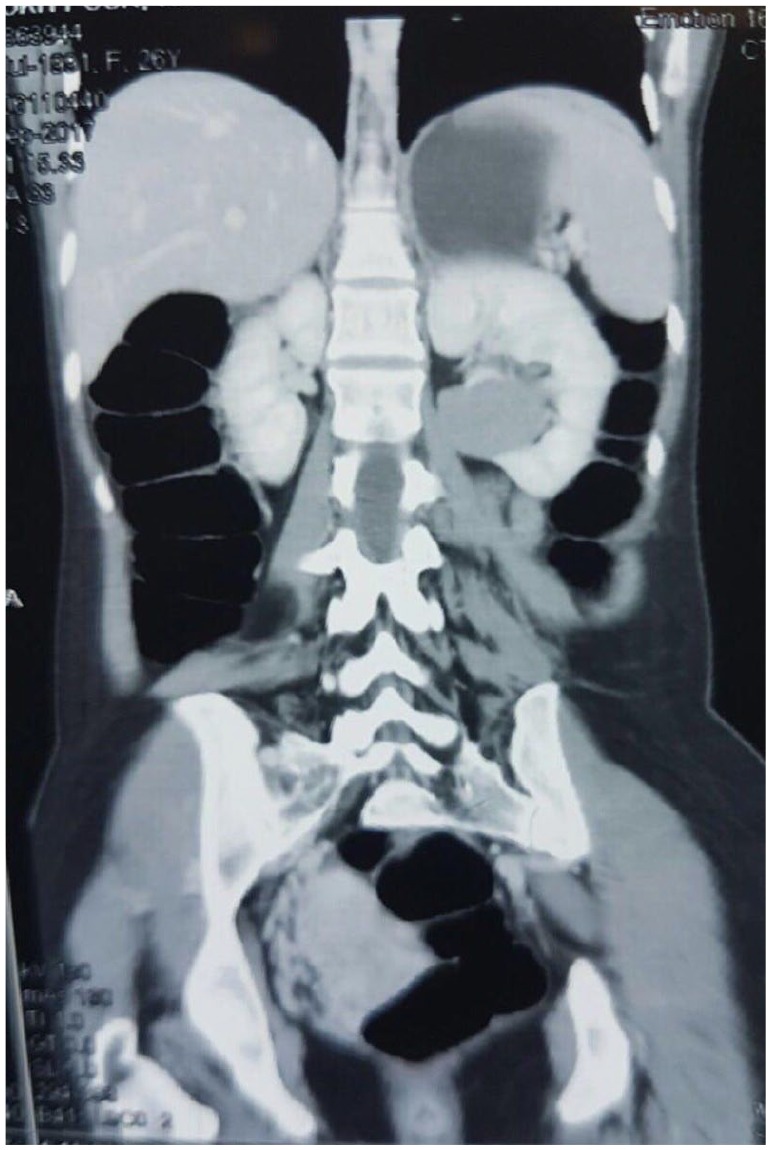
Abdominopelvic spiral CT scan: a 38 mm × 30 mm × 43 mm hypodense solid mass
